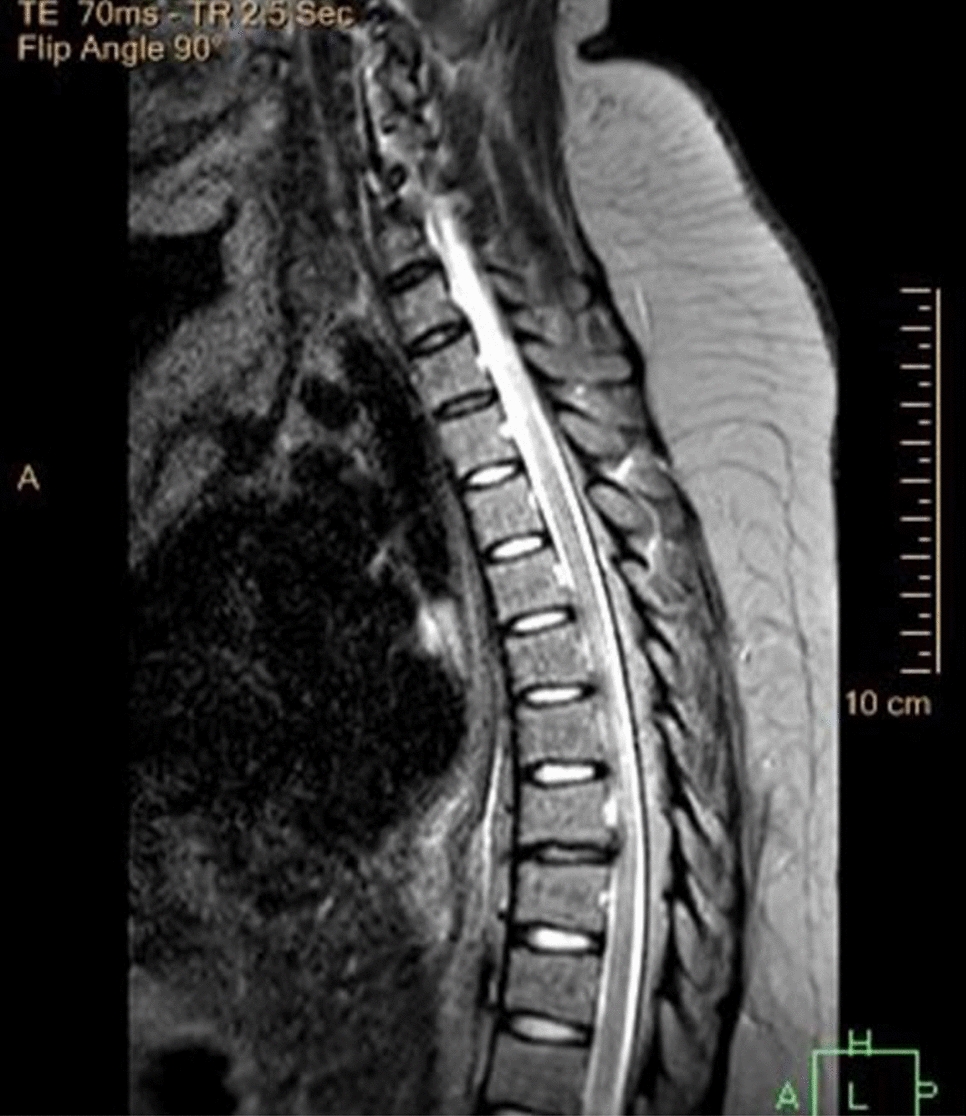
MRI spine technique: T2W1, T1W1 (pre- and post-contrast) sagittal, axial view thoracic area. The apparent loss of cord volume, more prominent at T5 to T7. Associated bilateral paramedian focal, linear T2W/STIR hyperintensity dorsal cord from the in these regions
